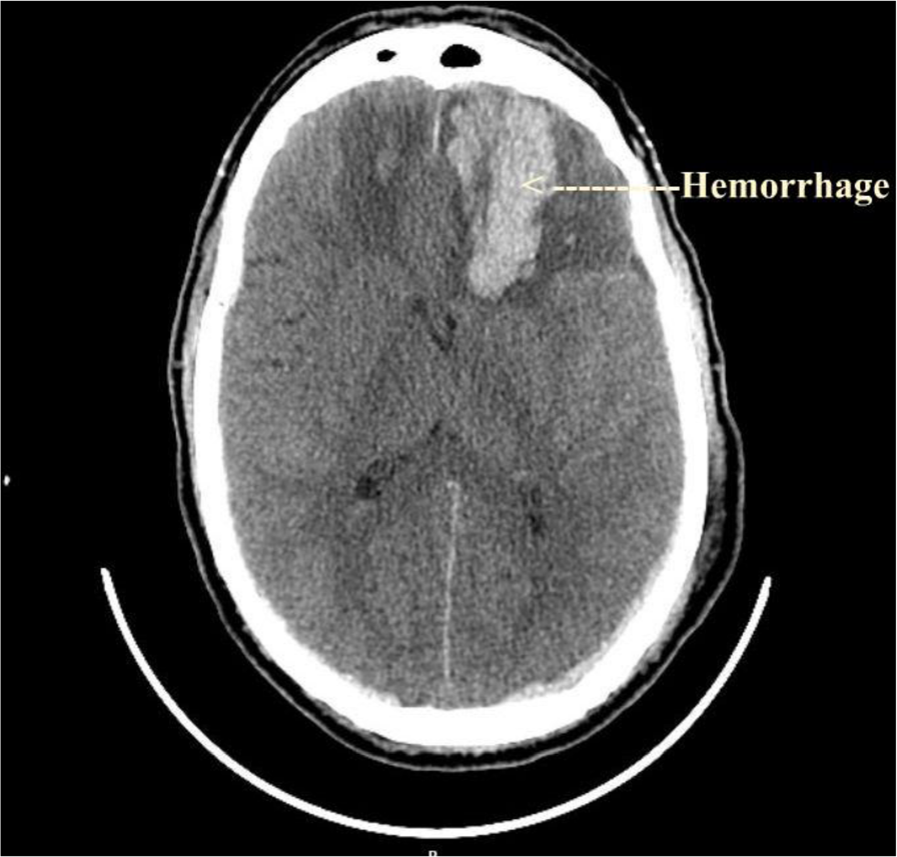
CT scan brain image of CASE 2 at initial presentation in hospital showing acute intraparenchymal hemorrhage in the frontal lobe area. This case suggests severity of presentation.
